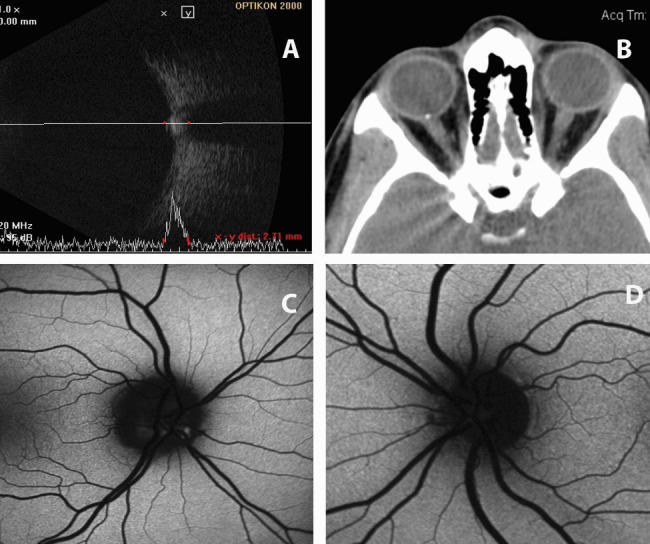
A and B ultrasound scans of the right eye showing a highly reflective area at the optic nerve head, compatible with drusen (A). CT scan of the orbits showing a bone density area at the optic nerve head of the right eye (B). Auto-fluorescence fundoscopic images of the right (C) and left (D) eyes, showing fluorescent bodies at the optic nerve head of the right eye, compatible with drusen
